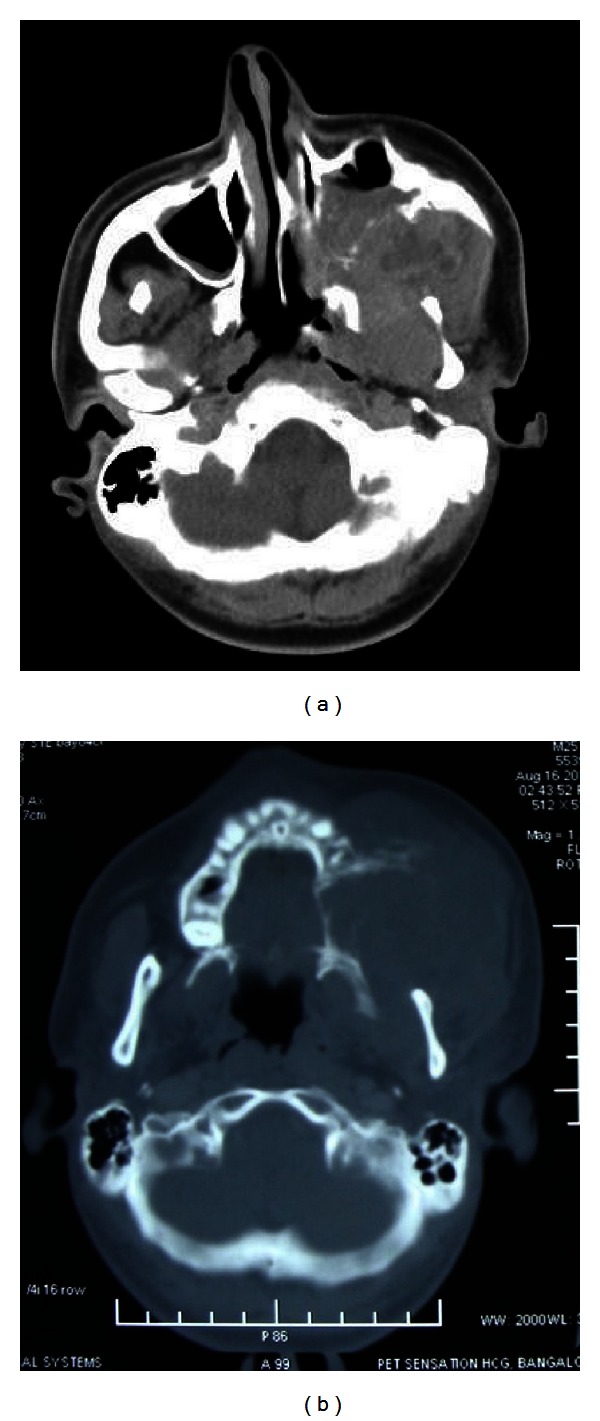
CT showing the extent of the lesion.
